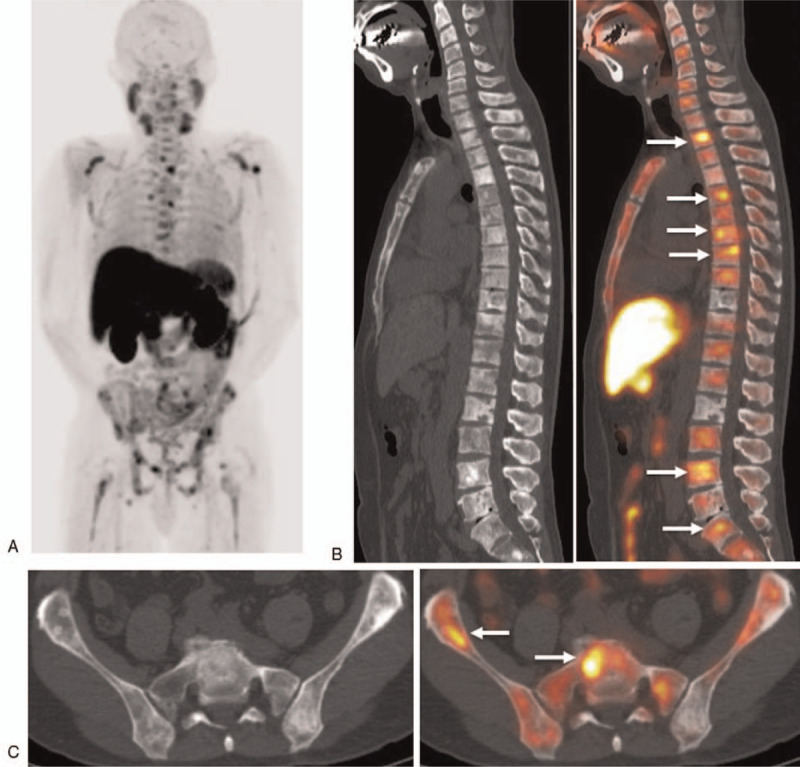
Forty-seven-year-old man with PSA level of 13.41 ng/mL who underwent hormonal therapy and docetaxel for prostate cancer (cT4N1M1b, initial PSA 180 ng/mL, Gleason score 5 + 5), in whom choline PET/CT could discriminate viable progressive osteoblastic bone metastasis from benign osteoblastic change induced by the treatment effect. (A) Maximum intensity projection (MIP) from ^11^C-choline PET/CT image shows abnormal ^11^C-choline uptakes in the spine, pelvic bone, scapula, and femur. (B) Sagittal ^11^C-choline PET/CT image shows multiple osteoblastic lesions in the whole spine with no ^11^C-choline uptakes, reflecting treatment-induced sclerosis during the treatment course and several abnormal ^11^C-choline uptakes in the thoracic (Th2,5,7,8) lumbar spine (L5), and sacral (S1) spine sclerosis (arrows), suggesting viable tumors. (C) Axial ^11^C-choline PET/CT shows several osteoblastic lesions in the sacral spine (S1) and ilium with no ^11^C-choline uptakes, reflecting treatment-induced sclerosis during the treatment course and several abnormal ^11^C-choline uptakes in the right ilium and S1 sclerosis (arrows), suggesting viable tumors.
